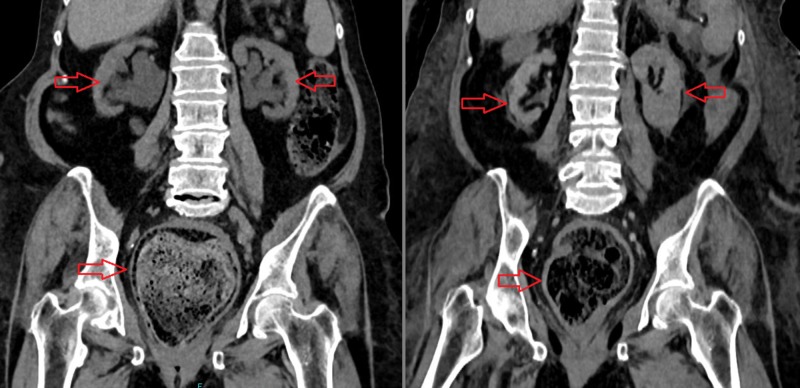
Non-contrast CT scan - coronal view At the time of admission (left): dilated and fecaloid-filled rectum and colon and bilateral hydroureteronephrosis in the urinary system (red arrows) can be seen. Two days later (right): after rectosigmoidal lavage, a decrease in the amount of fecaloid in the rectum and regression in the degree of hydroureteronephrosis (red arrows) can be observed CT: computed tomography
